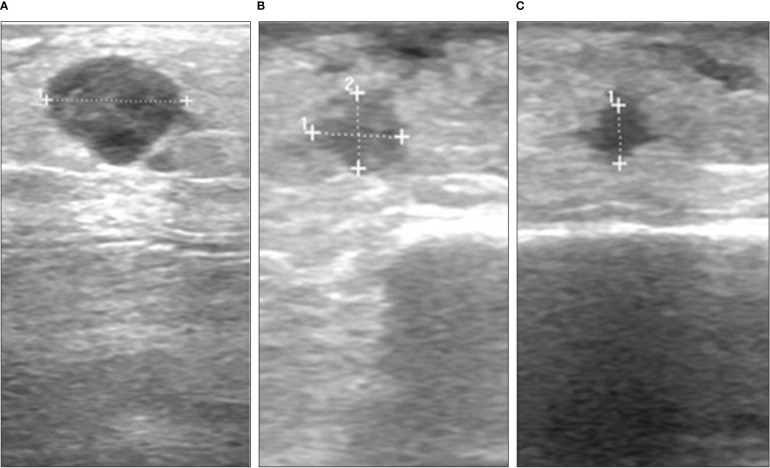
Course of a subcutaneous melanoma metastasis under combination of MEK inhibitor and T-VEC in case 1. Ultrasound images from (A) April 2017, (B) April 2018 and (C) January 2019.
